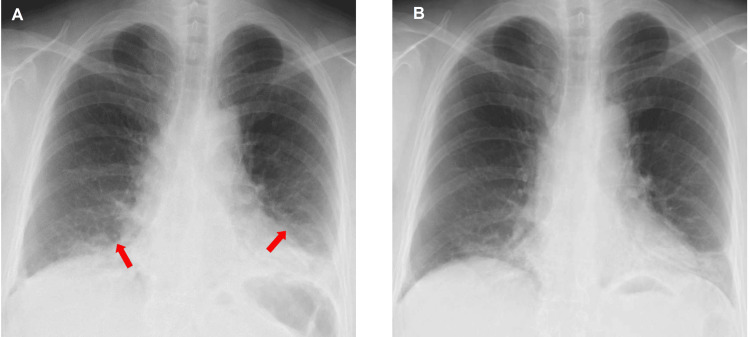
Chest radiography of the patient before and after treatment (A) Chest radiography at the first visit: attenuation of the inferior lung observed; (B) Chest radiograph on the day of discharge: improved attenuation of the inferior lung.
